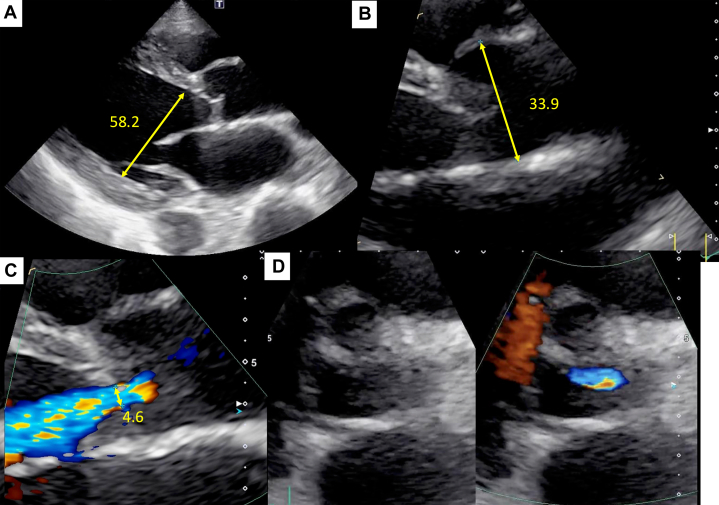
Transthoracic Echocardiography at Admission Transthoracic echocardiography shows moderate-to-severe aortic regurgitation. Left ventricular systolic function was significantly impaired, with an ejection fraction of 21.1% by the Teichholz method and 8.7% by the modified Simpson’s method. The left ventricle is enlarged, with end-diastolic and end-systolic dimensions of 58.2 mm and 52.5 mm, respectively (A, B). The vena contracta width is 4.6 mm (C, D).
